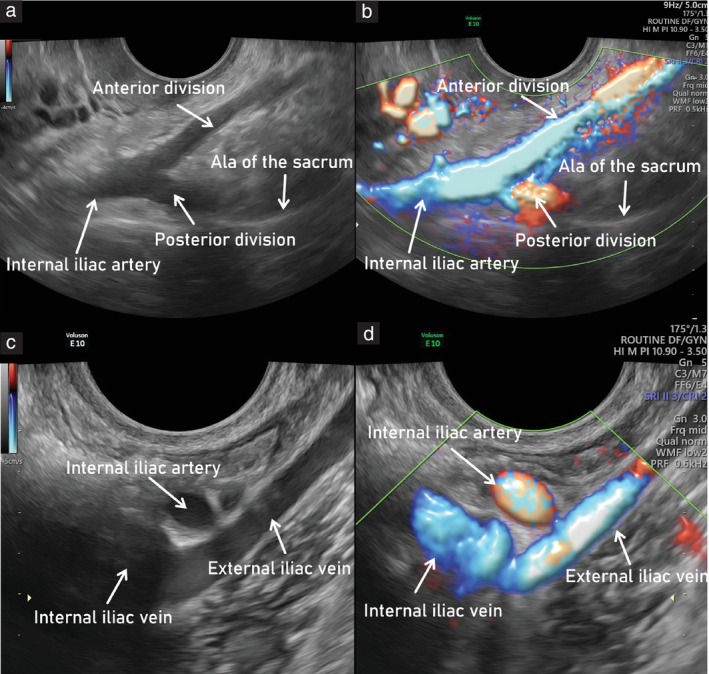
Main vascular bifurcations in the pelvis (left side). Ultrasound images showing: (a,b) branching of internal iliac artery into its anterior and posterior parts (forming a V shape) on grayscale (a) and power Doppler (b) assessment; and (c,d) interiliac bifurcation, showing external and internal iliac veins, on grayscale (c) and power Doppler (d) assessment. To obtain the entire course of the external iliac vessels, the probe should be rotated 90° from transverse (a,b) to longitudinal (c,d) plane.
